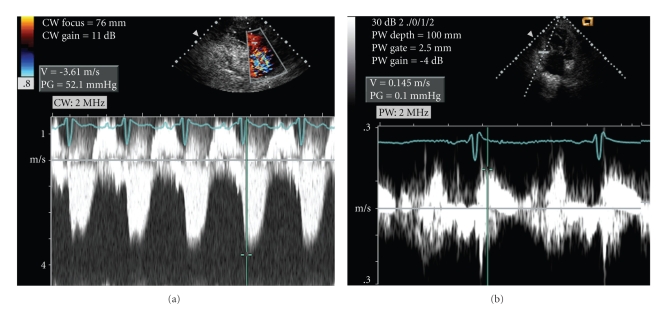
(a) Continuous wave Doppler profile of tricuspid regurgitation in the parasternal long-axis view. The tricuspid valve regurgitant velocity is 3.61 m/s. Using the modified Bernoulli equation (Δ*P* = 4*V*
